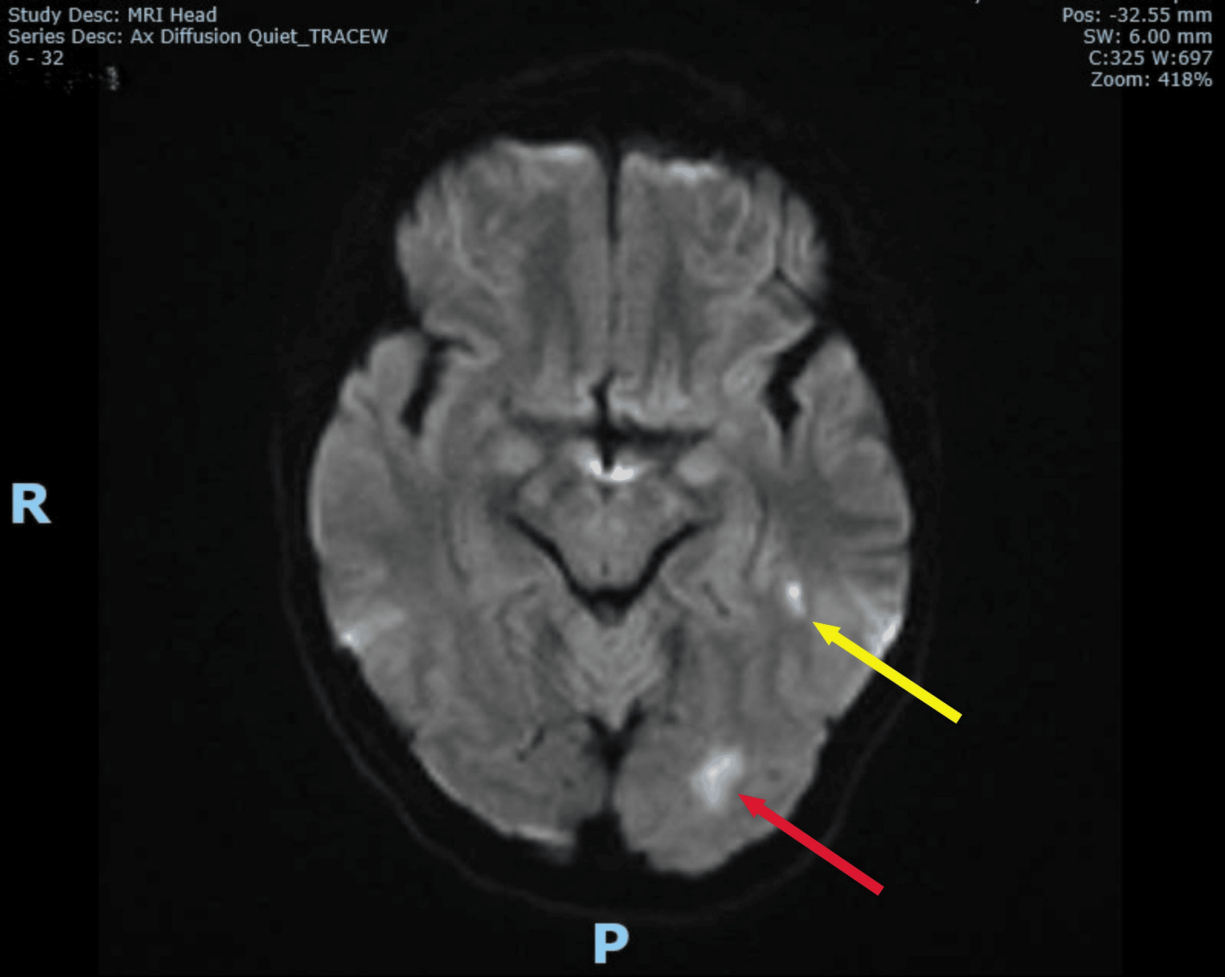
MRI brain DWI showing infarcts at posterior left temporal lobe (yellow arrow) and inferior left occipital lobe (red arrow) MRI: magnetic resonance imaging DWI: diffusion-weighted imaging
